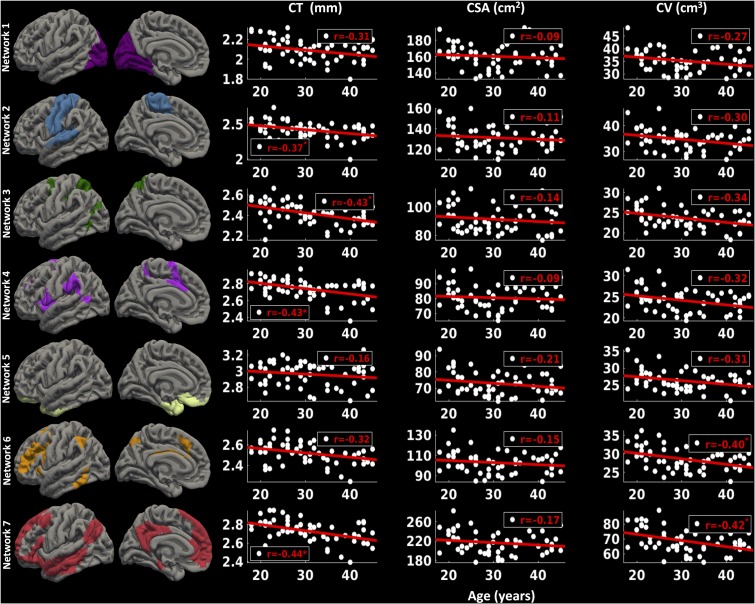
Network-wise age-related changes in cortical measures for left hemisphere. Linear plots showing significant or trend toward significant reduction in cortical thickness (CT in mm) (^∗^*p* < 0.05, corrected for multiple comparisons), non-significant reduction in cortical surface area (CSA in cm^2^) and significant or trend toward significant reduction in cortical volume (CV in cm^3^) (^∗^*p* < 0.05, corrected for multiple comparisons) for seven functional networks on left hemisphere.
